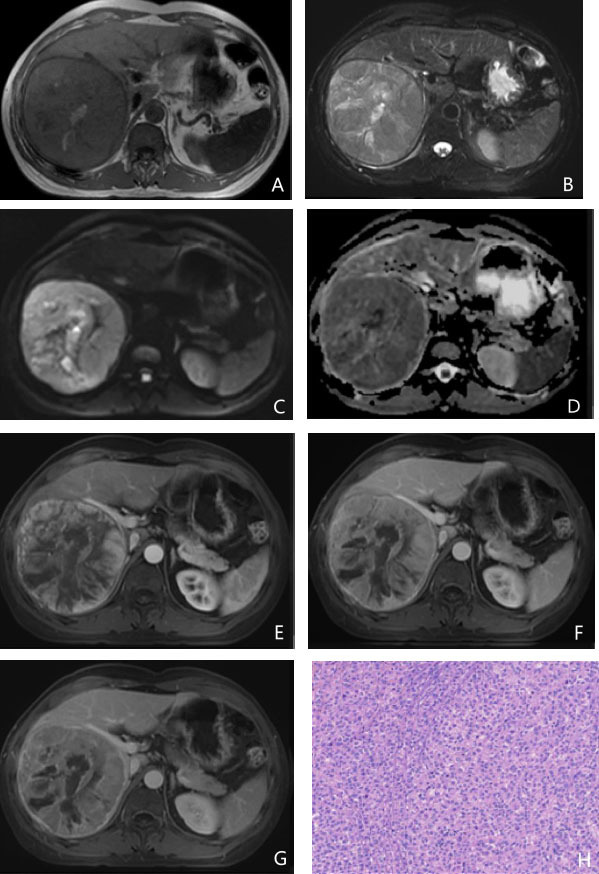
A 49-year-old male HCC patient. A: T1WI showed low signal as well as high signal in the center. B: T2WI showed a heterogeneous high signal. C: DWI showed a high signal. D: ADC showed a low signal. E, F, G: After enhancement, the lesion showed diffuse heterogeneously high enhancement in the arterial phase and decreased enhancement in the portal venous and delayed phases, with capsular enhancement. H: Pathological observation (10×) showed that tumor nuclei were large and had less surrounding fibrous tissues.
